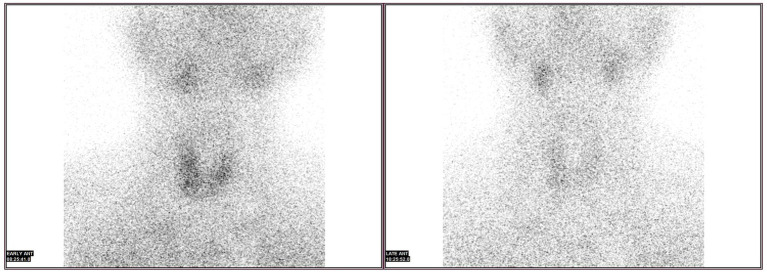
SPECT/CT showed a focal area of increased radiotracer uptake at the inferior pole of the right thyroid lobe, suggestive of a hyperfunctioning parathyroid gland.
